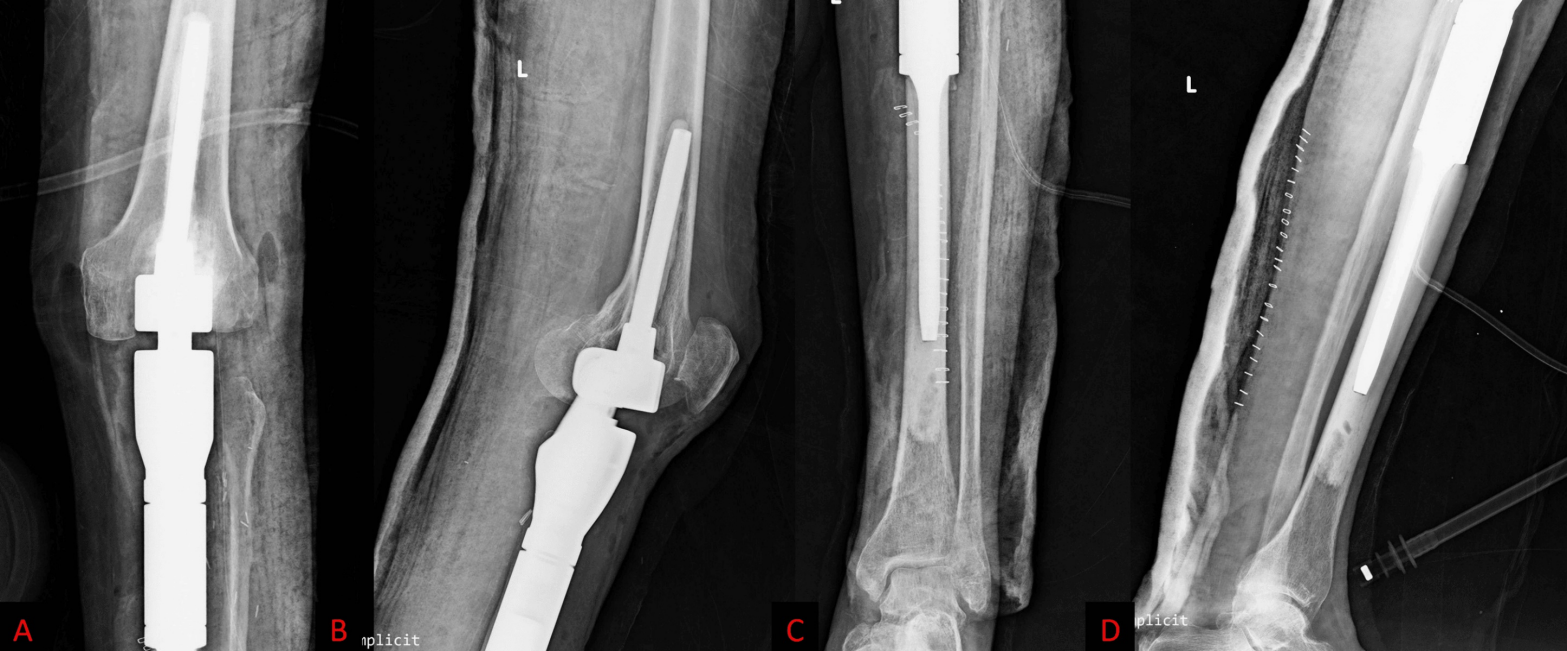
Postoperative X-ray: (A) AP view and (B) lateral view of the knee and (C) AP view and (D) lateral view of the leg reveal a metallic endoprosthesis in place after wide excision of the lesion in the proximal tibia. AP: anteroposterior
